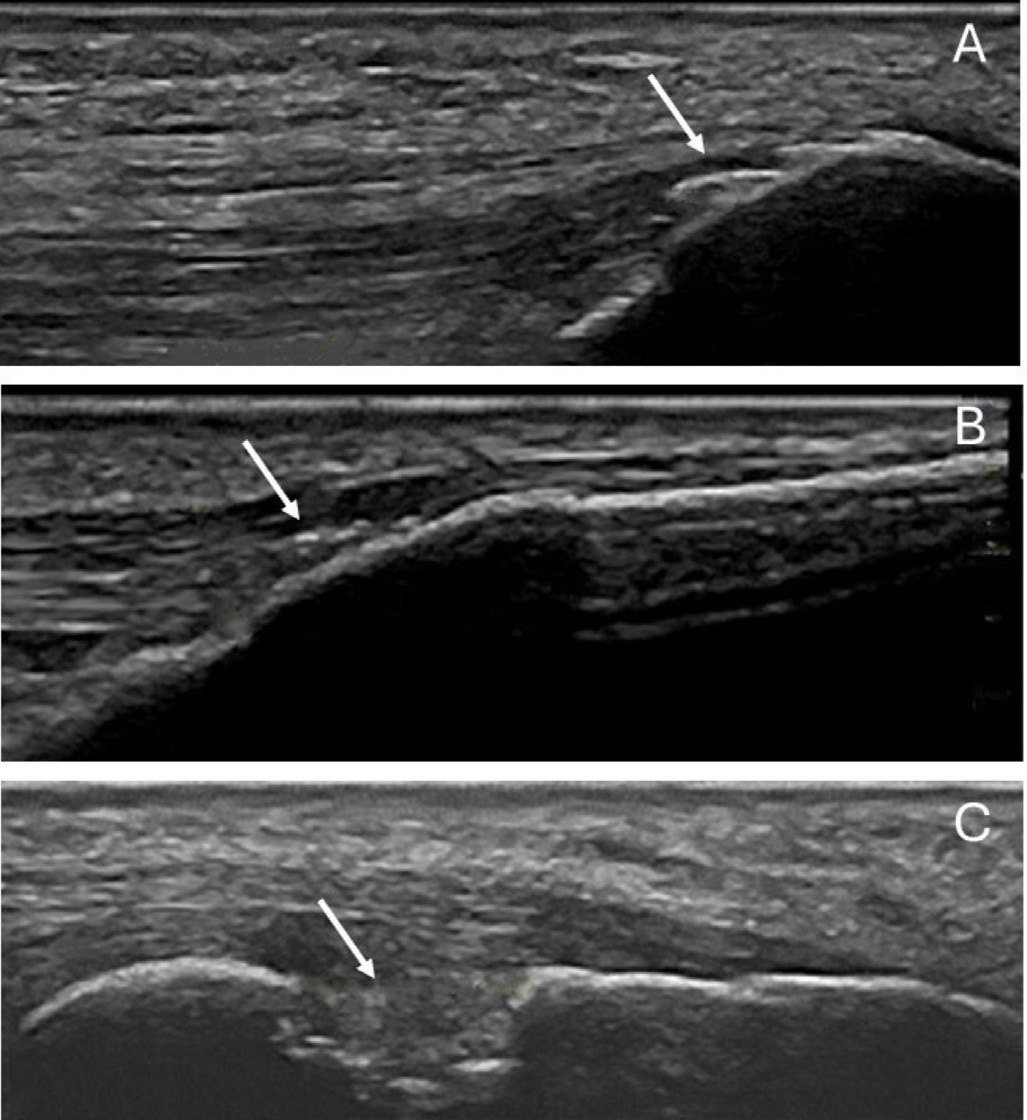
Ultrasound images of (A) enthesophytes at the quadriceps tendon insertion on the patella. (B) Calcifications at the patellar ligament insertion on the tibia. (C) Erosion at the Achilles tendon insertion on the calcaneus.
